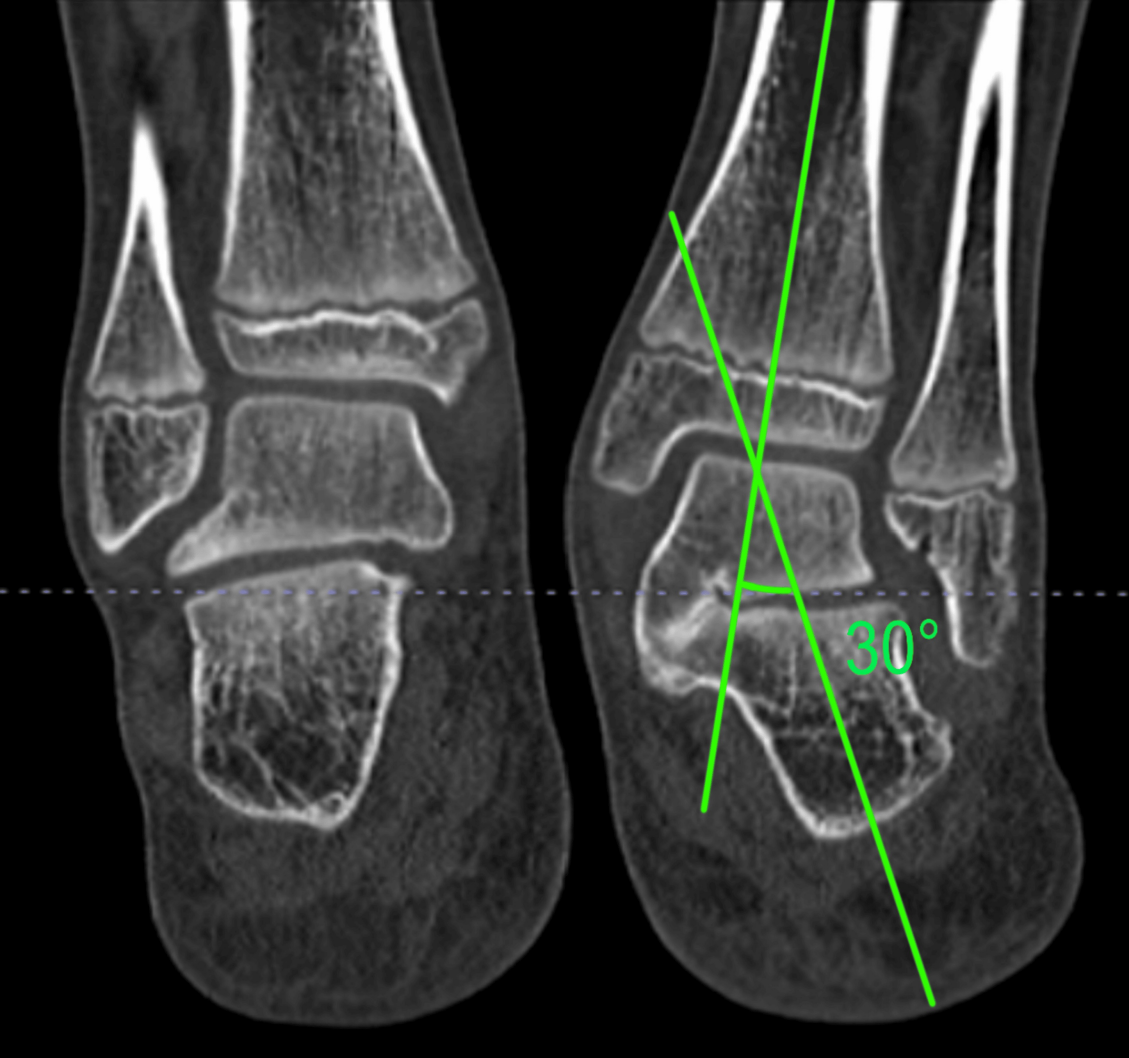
Measurement of hindfoot valgus on coronal reformats of CT. The left foot was normal, while the right foot exhibited flatfoot and a talocalcaneal coalition (TCC).
